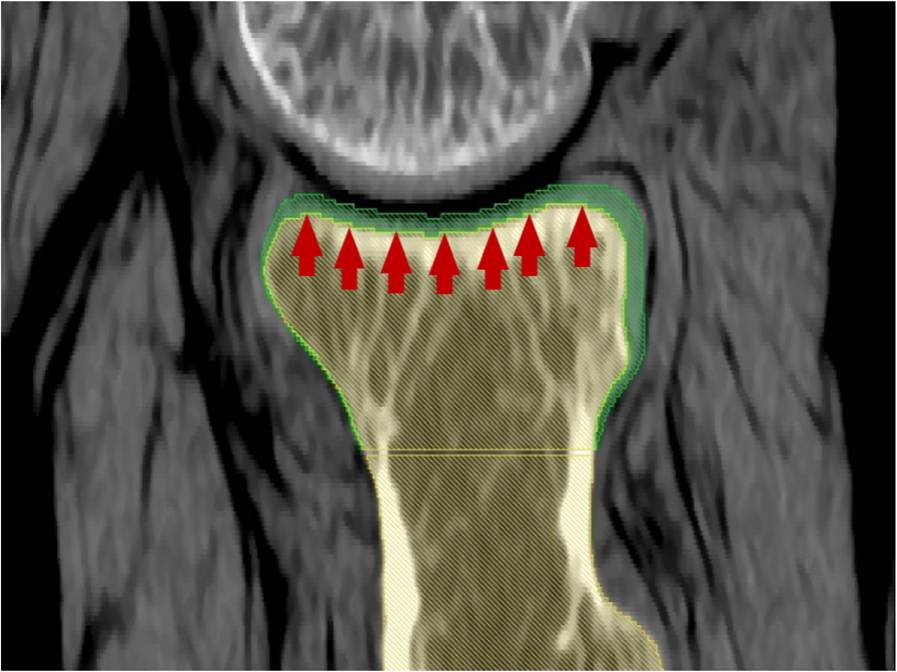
The cartilage-reproducing image reconstruction method of CT image at radiocapitellar joint. Translate the articular portion toward the capitellum until a reformed border  touches half the distance between both subchondral surfaces
